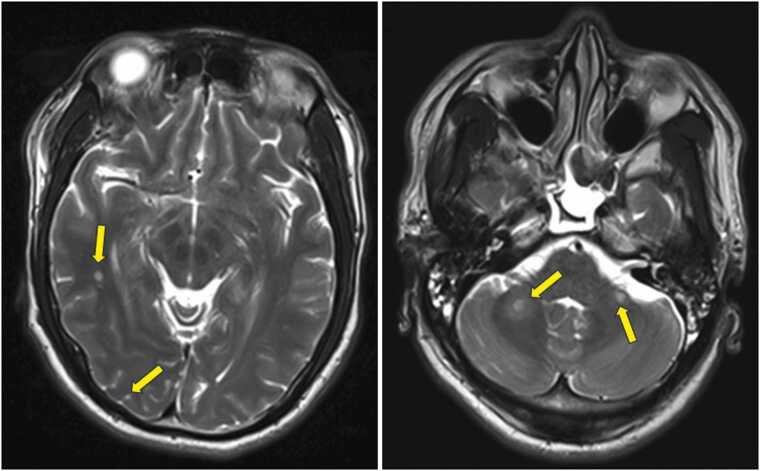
MRI brain showed multiple small foci (yellow arrows) of restricted diffusion, suggestive of central-embolic microinfarcts.
